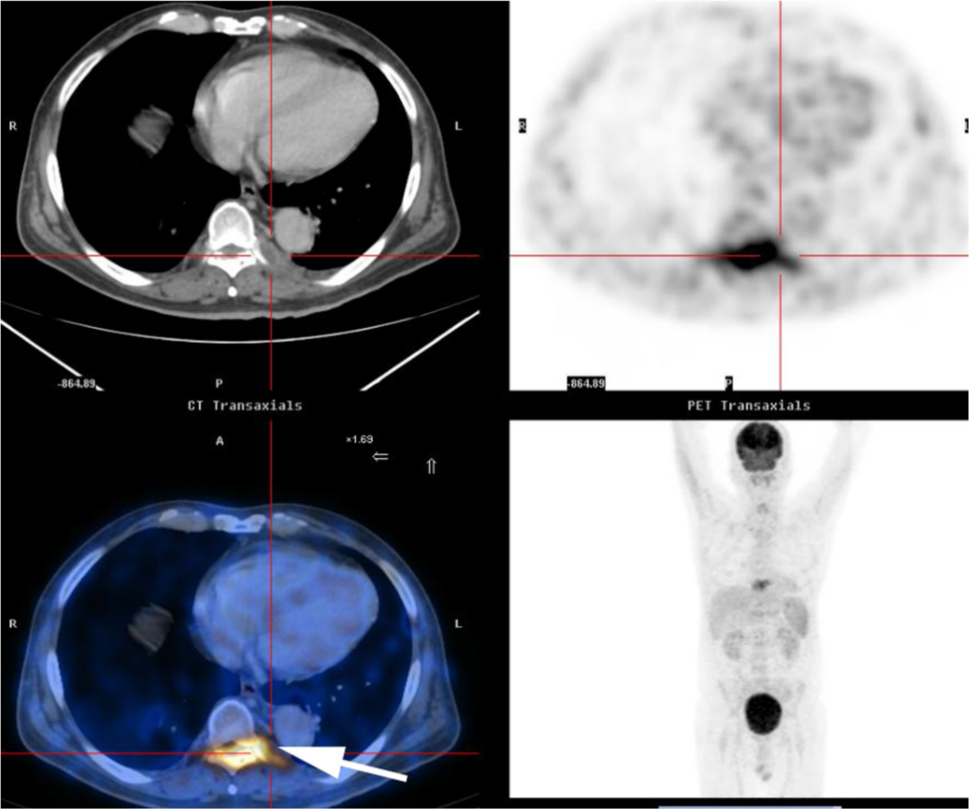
Positron emission tomography-computed tomography scan of the total body. Radionuclide concentration (single-headed arrow) was detected at the vertebral arch of T9
